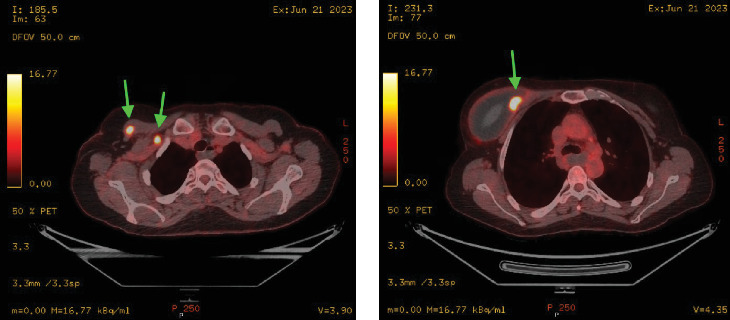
PET-CT, fused axial series, images 63 and 77, respectively (21/06/23). Images show biopsy-proven BIA-ALCL with abnormal avid sub-pectoral nodes (green arrows).
